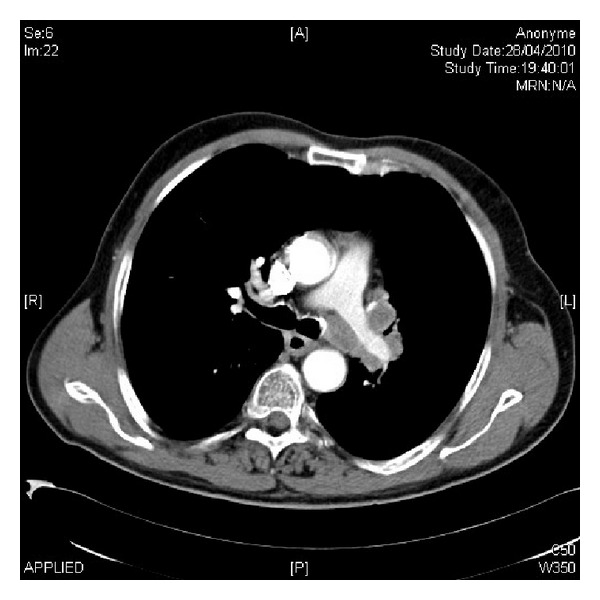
Thoracic imaging by computed tomography (CT) revealed a left hilar mass with associated left hilar and mediastinal lymphadenopathy.
